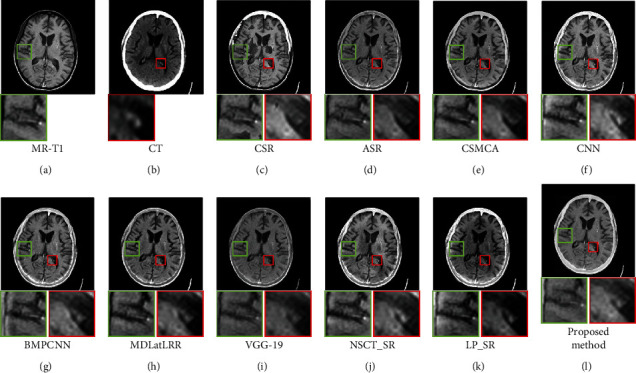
Comparison of the fusion results in the first set images. (a) MR-T1. (b) CT. (c) CSR. (d) ASR. (e) CSMCA. (f) CNN. (g) BMPCNN. (h) MDLatLRR. (i) VGG-19. (j) NSCT_SR. (k) LP_SR. (l) Proposed method.
